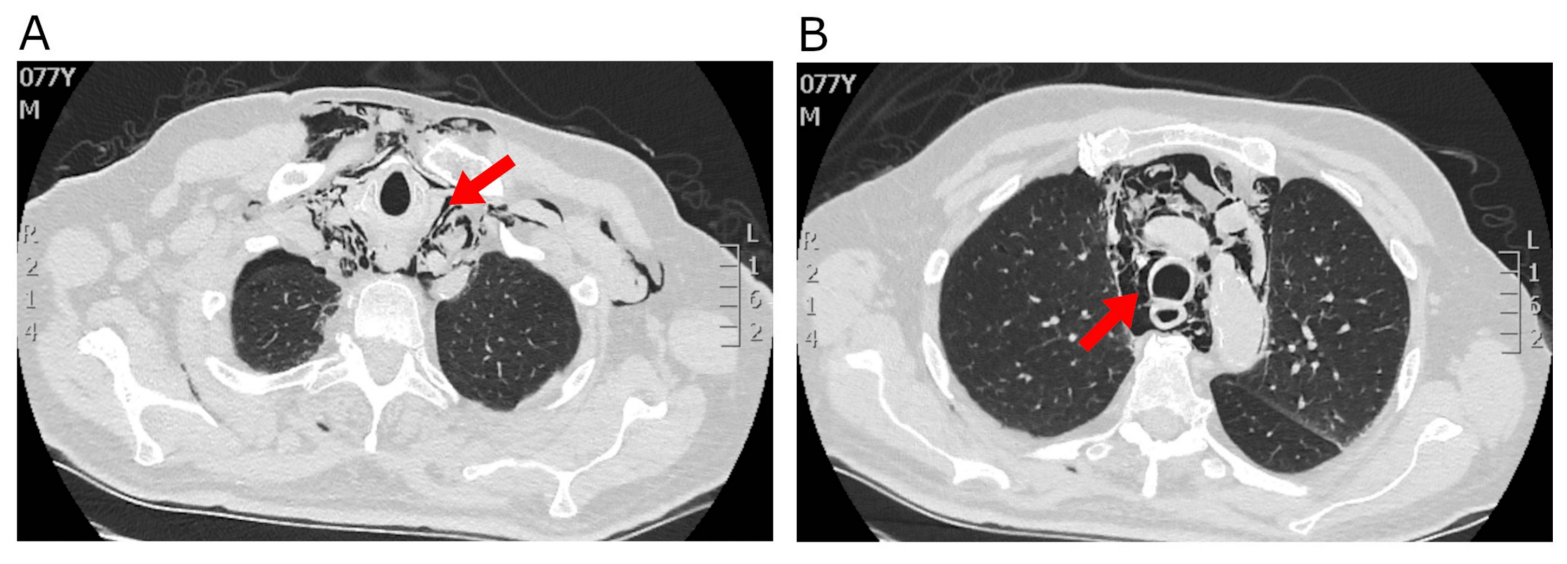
CT of the chest showing pneumomediastinum (red arrows) of a large extent (A and B)
